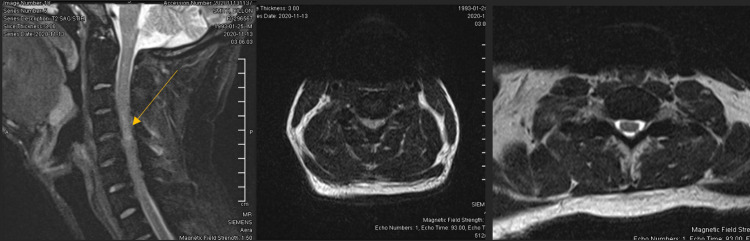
T2 cervical MRI (left) showing cord edema (arrow) at C4-C5 and cord compression on the axial view (center). Note the spinal cord compression and lack of CSF fluid surrounding the cord (center) when compared to the lower cervical spine (right). CSF - Cerebrospinal fluid
